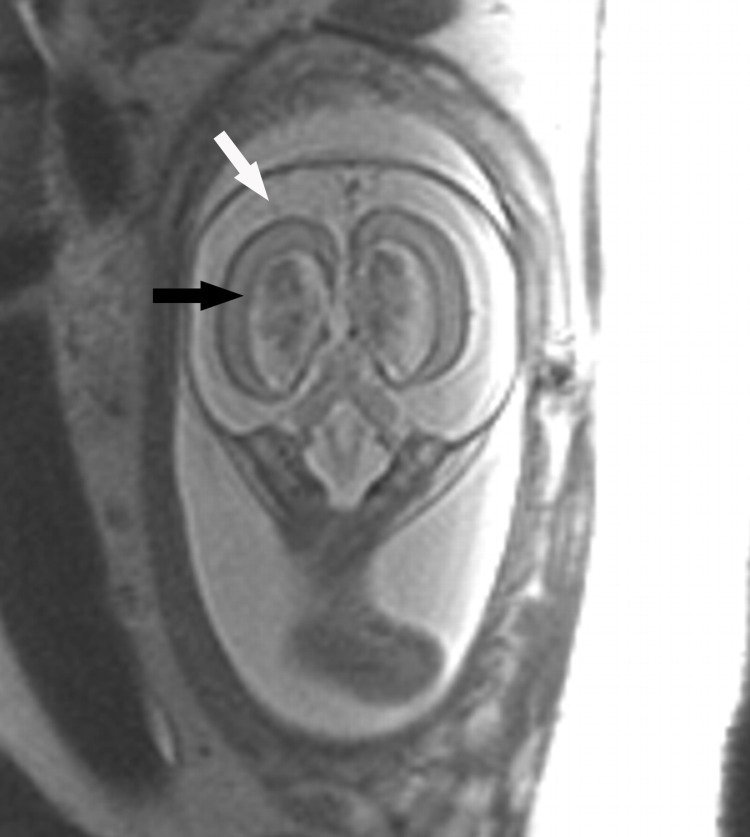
Coronal T2-weighted single-shot fast spin-echo of the fetal brain at 20 weeks and 6 days. Coronal T2-weighted single-shot fast spin-echo of the fetal brain at 20 weeks 6 days demonstrates enlargement of the lateral ventricles (black arrow), filled with prominent choroid plexus. Note also made of enlarged bilateral extra-axial spaces (white arrow). Parenchymal thinning was present, likely secondary to ventriculomegaly.
